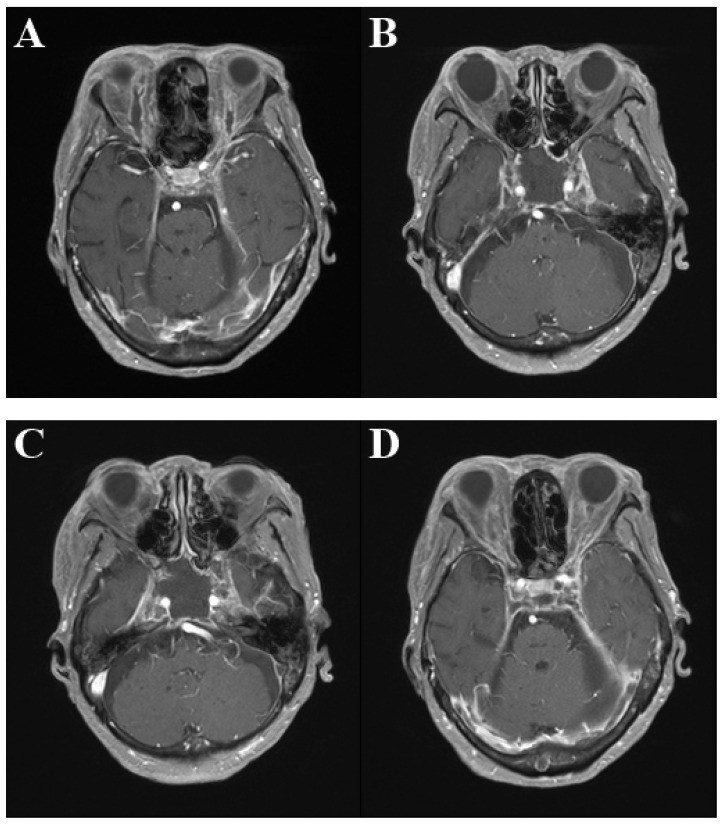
Brain magnetic resonance imaging with contrast enhancement. (A) Bilateral intraluminal sinus thrombosis and enlarged superior orbital vein; (B) filling defects in the bilateral cavernous sinus; (C,D) T1-weighted image with contrast revealed low signal intensity within transverse sinus and sigmoid sinus.
